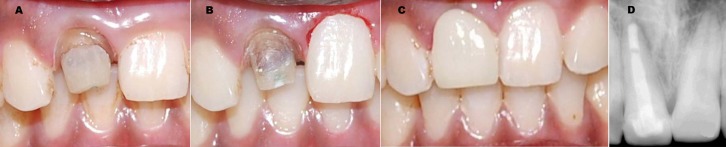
A: Clinical photograph after post cementation. B: Gingivectomy of maxillary left central incisor. C: Clinical photograph after metal-free ceramic crown cementation. D: radiograph after six months showing complete healing of periapical lesion.
